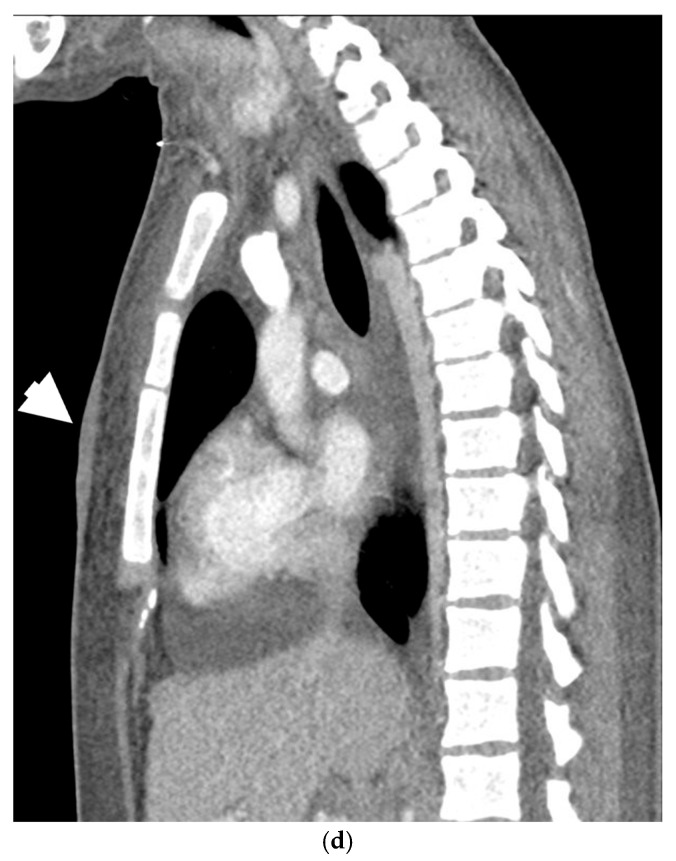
17-year-old male with a history of acquired immune deficiency syndrome and Kaposi’s sarcoma presents with respiratory distress. Coronal CT image in lung window (a) shows right-greater-than-left multifocal flame-shaped pulmonary opacities (dashed black arrows) consistent with pulmonary Kaposi’s sarcoma. Additional coronal and axial CT images in lung window (b,c) reveal a small right pneumothorax (open black arrow). Sagittal CT image in soft tissue window (d) demonstrates focal skin thickening in the anterior chest wall (white arrowhead), also in keeping with Kaposi’s sarcoma.
